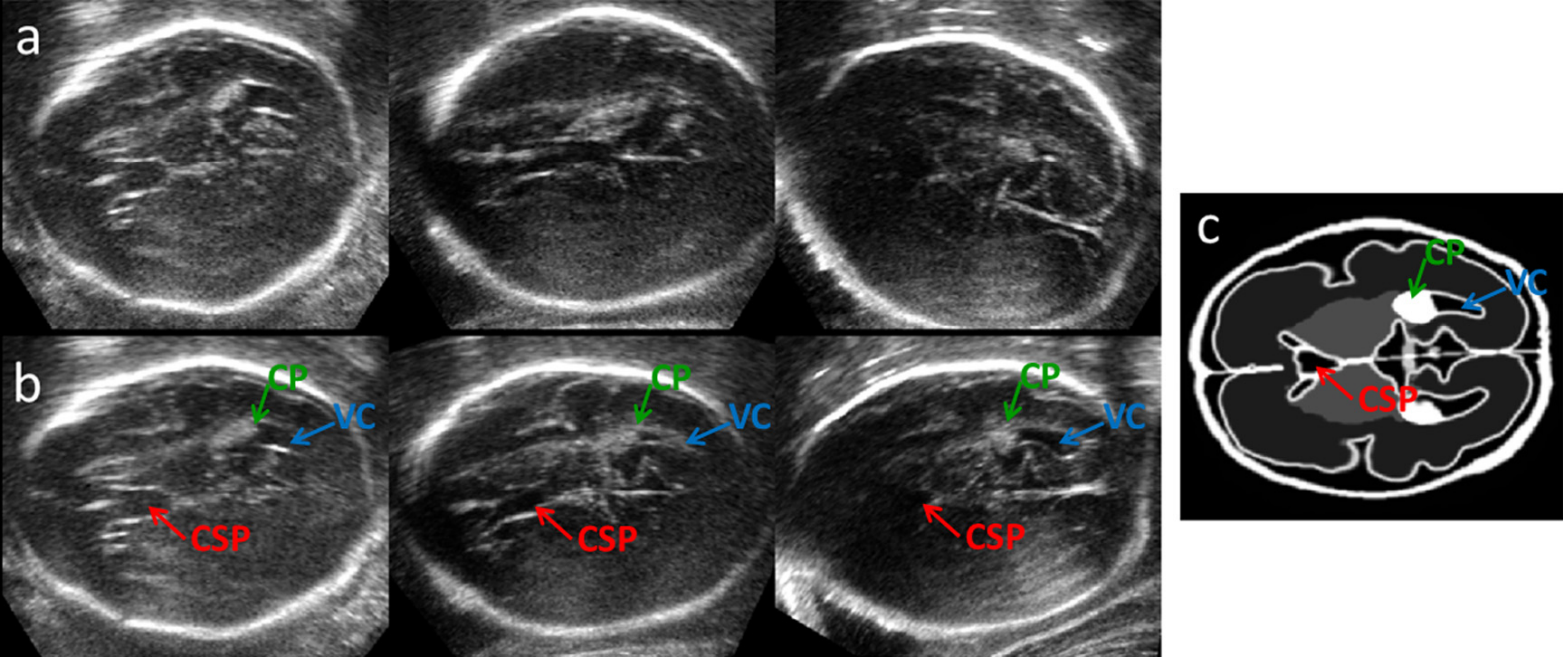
A 2D view of 3D US of three fetuses with GA around 29 weeks before (a) and after (b) alignment with the MRI. After alignment the view shows the transventricular plane in all three cases. Compare to pseudo US image (c). Note that CP, CSP and VC are visible, while cerebellum does not appear, as expected in transventricular plane.
